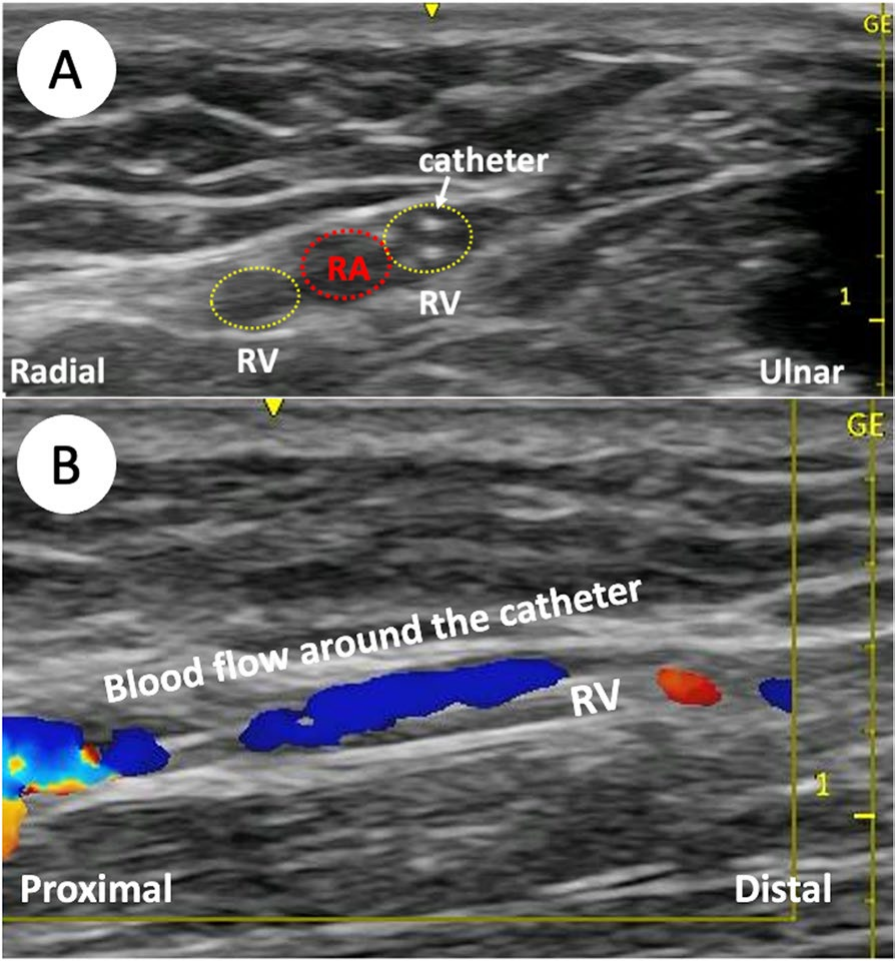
Ultrasound short- (A) and long-axis (B) view of the right forearm in Case 2
